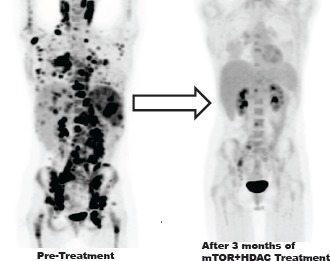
FDG PET/CT scans showing metabolic response to therapy Response in the 26 year old female with classic Hodgkin's lymphoma with sirolimus and vorinostat after 6 lines of therapy. Pre-treatment scans show extensive lymphoma. Scans after 3 cycles of therapy show an exceptional response and decreased SUV activity.
